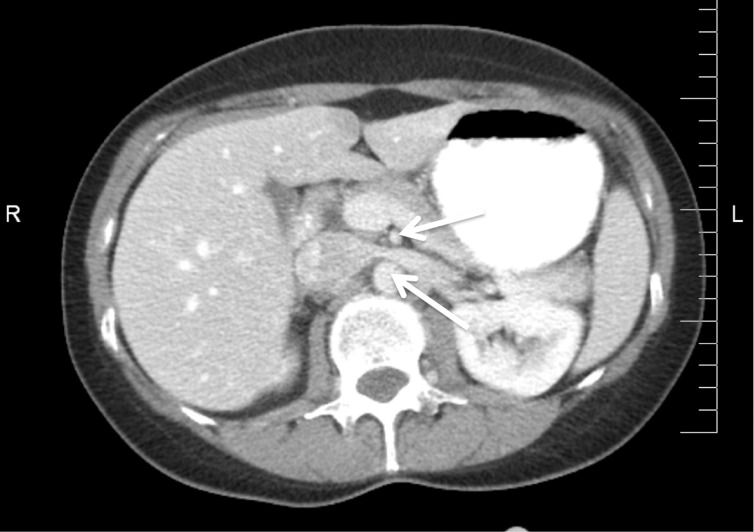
Axial CT of the abdomen Relationship of the superior mesenteric artery (upper arrow) and aorta (lower arrow)
